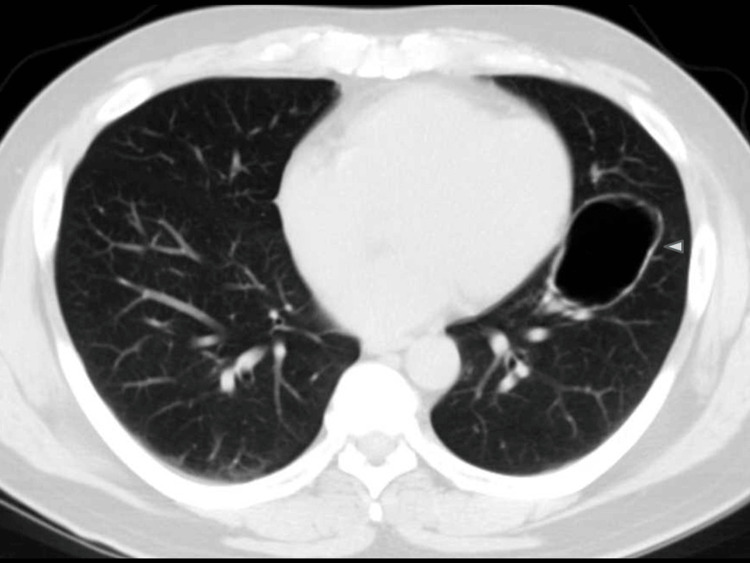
Historical chest CT. Chest CT performed over 10 years prior demonstrates a cystic lesion in the lingular segment of the left upper lobe (arrowhead), indicating a longstanding structural abnormality.
